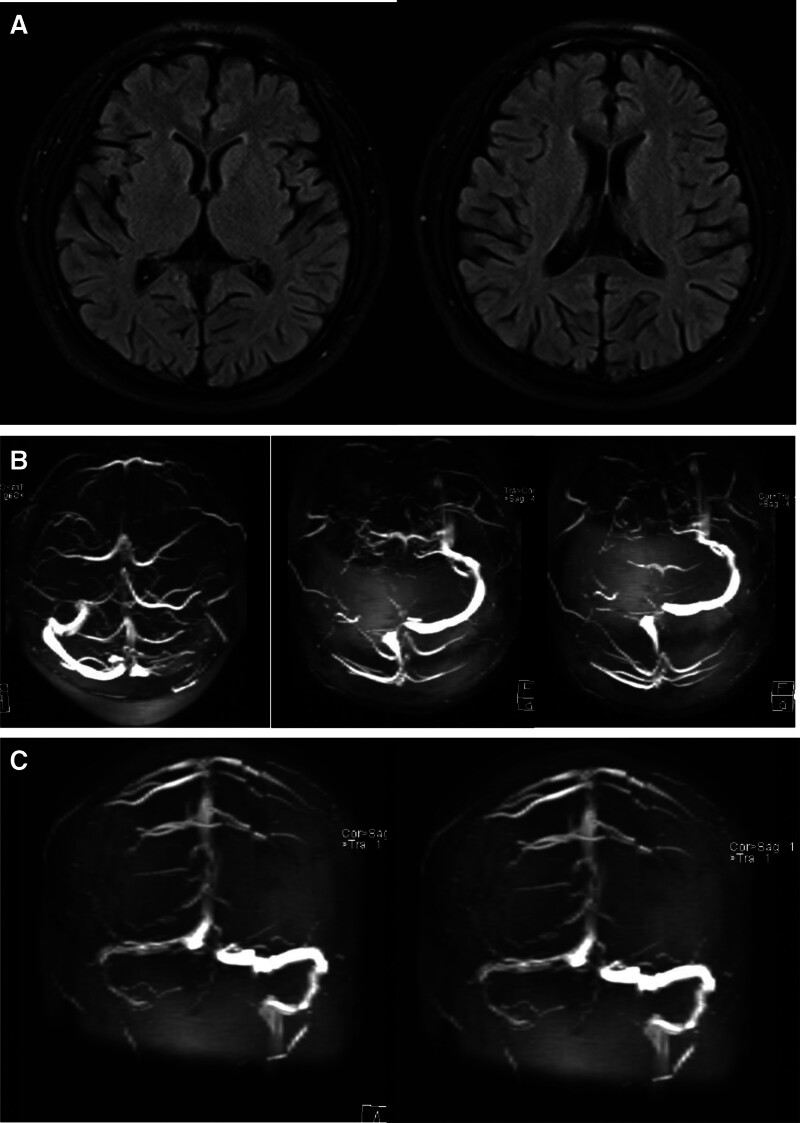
(A) Brain MRI (2021): mild brain atrophy, showing slight reduction in brain atrophy compared to the previous scan in 2016. (B) (2021) MRV: there was a significant likelihood of venous thrombosis in the right transverse sinus, sigmoid sinus, and the lumen of the internal jugular vein. The enhancement of the superior sagittal sinus lumen was locally diminished, which does not exclude the possibility of local thrombosis or slow venous. (C) Follow-up MRV after treatment: the right transverse sinus and superior sagittal sinus lumen show improvement compared to previous scans, while the right sigmoid sinus and internal jugular vein appear largely unchanged. MRI = magnetic resonance imaging, MRV = magnetic resonance venous imaging.
